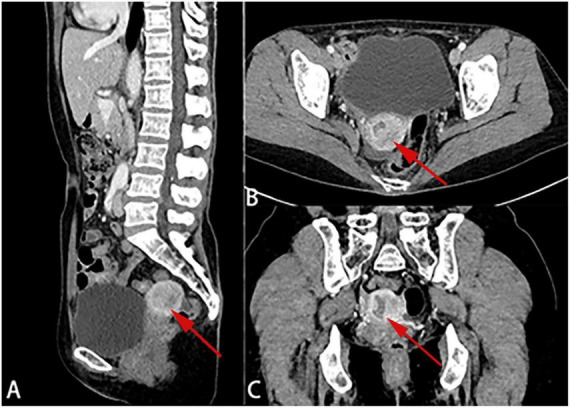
(A–C) Preoperative pelvic contrast-enhanced computed tomography (CT) demonstrated irregular thickening of the endometrium with heterogeneous enhancement.
